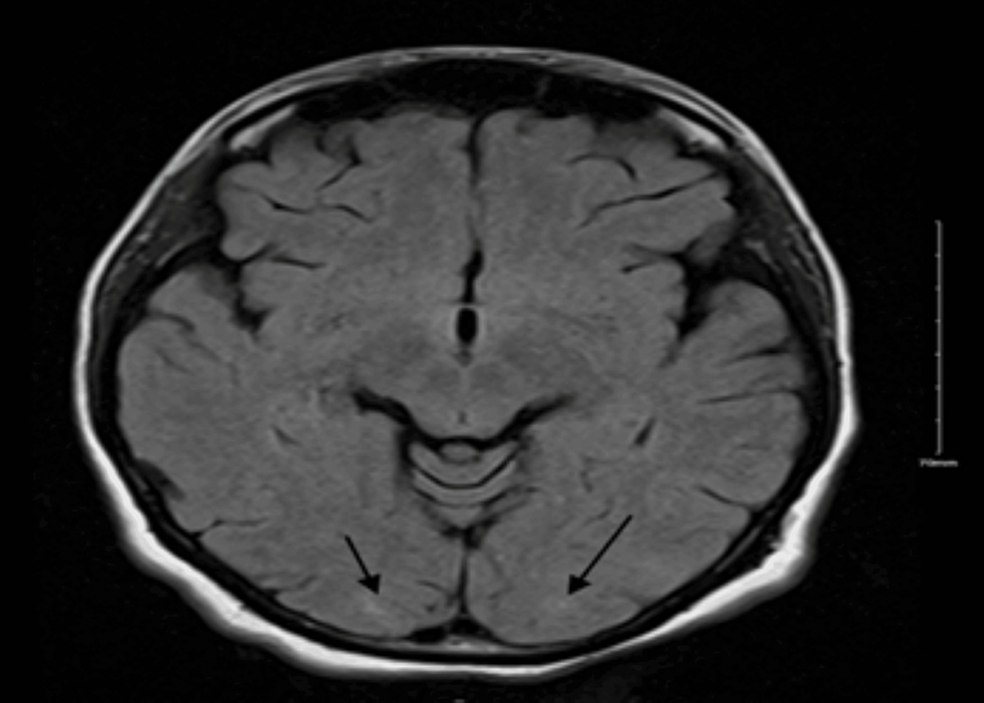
FLAIR MRI done one week after the initial showing a resolution of the high signal intensity changes FLAIR: fluid-attenuated inversion recovery
